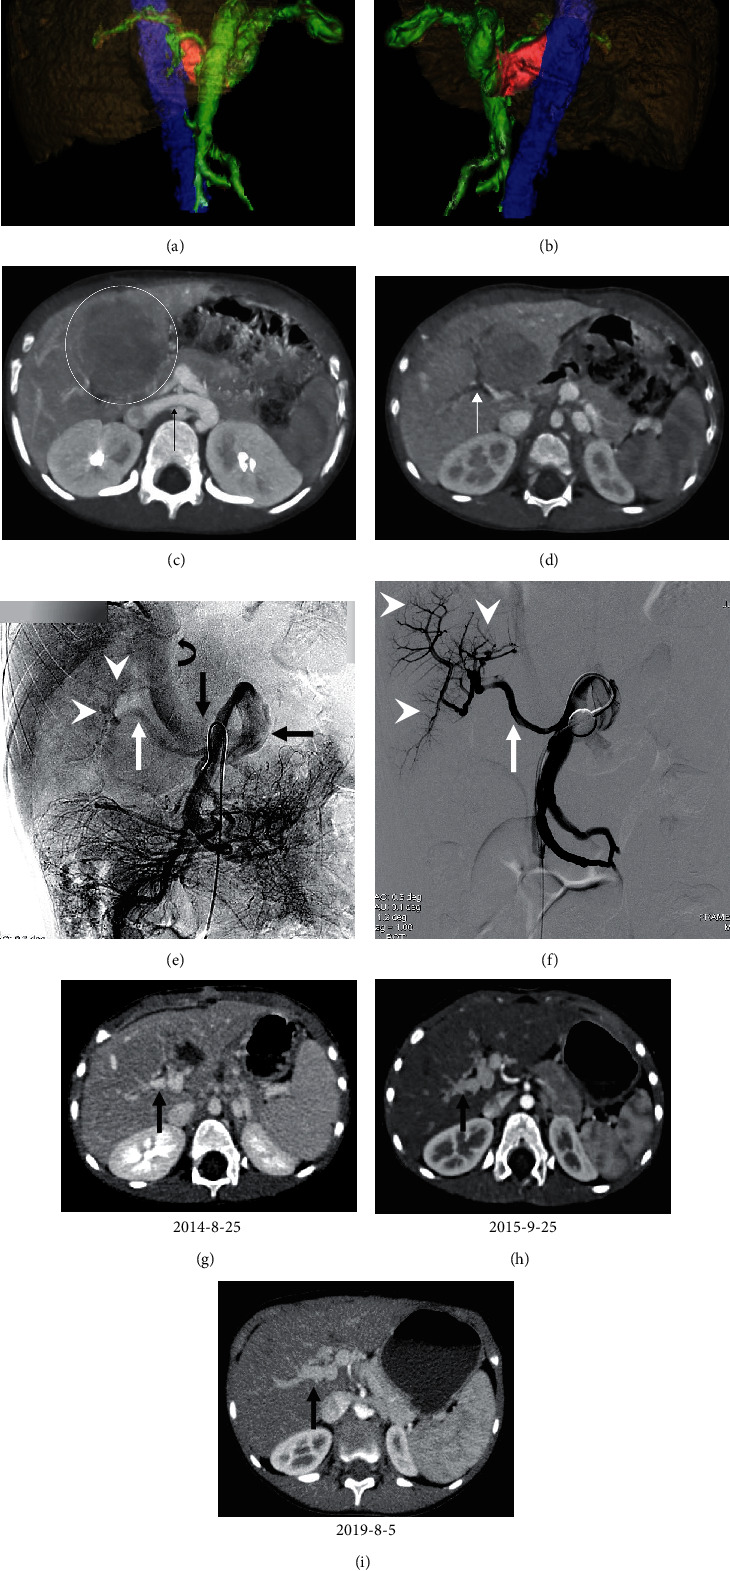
Images in a 3-year-old girl diagnosed with type II CEPS with hepatic encephalopathy (HE) who underwent surgical ligation due to large portal vein-IVC shunt. (a) Virtual reality (VR) images reconstructed from computed tomographic angiography (CTA) data prior to ligation. Anteroposterior view shows the portal vein and its branches (green), inferior vena cava (IVC) (blue), and the patent shunt communicating portal vein and IVC (red). (b) Posteroanterior VR image shows the portal vein and its branches (green), IVC (blue), and the portocaval shunt (red). (c) Axial CT image shows the large portal vein-IVC shunt (black arrow) and hepatic adenoma (circle) diagnosed by biopsy. (d) Axial contrast-enhanced CT image shows hypoplastic intrahepatic portal veins (white arrow) preoperative. (e) Indirect portal venography via the superior mesenteric artery (SMA) demonstrates venous outflow of the superior mesenteric vein (SMV) through the shunt (black arrow) drained into IVC (curved arrow), main portal vein (white arrow), and hypoplastic intrahepatic portal vein branches (arrowhead) which are visible. (f) Portal venography with balloon occlusion shows fine main portal vein (white arrow) and hypoplastic intrahepatic portal vein branches (arrowhead). The portal venous pressure (PVP) is 14.7 and 16.5 mmHg before and 15 min after balloon occlusion, respectively. (g) Contrast-enhanced CT images demonstrate the intrahepatic portal veins at 1 month after surgical ligation. (h) CT scan shows the intrahepatic portal veins at 12 months after surgical ligation. (i) CT scan shows the intrahepatic portal veins at 60 months after surgical ligation; the portal vein grows well over time.
